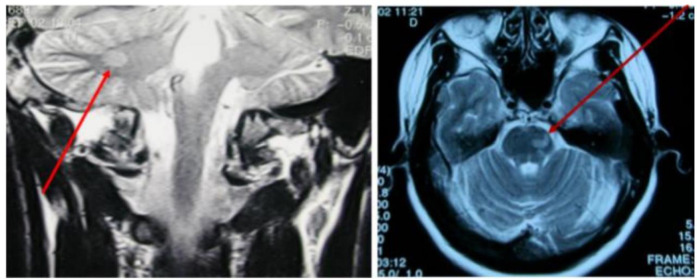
Infratentorial lesions on MRI.
